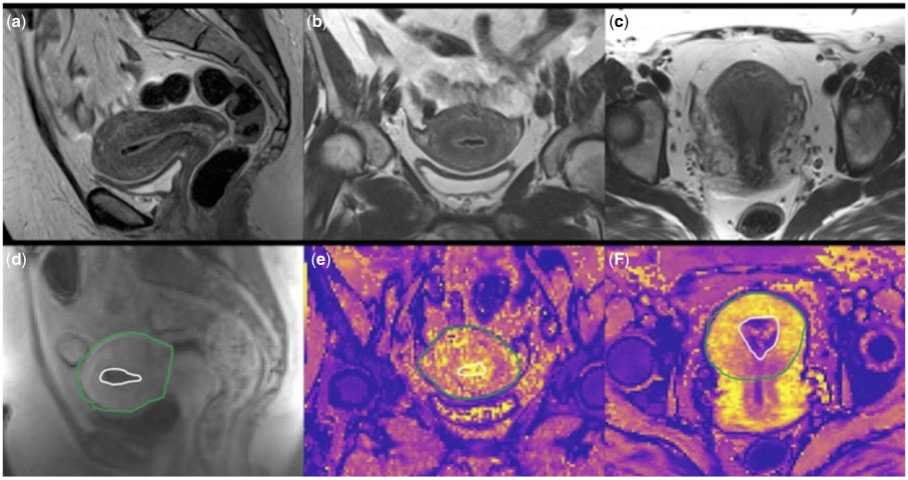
Example images and regions of interest. Top row: T2-weighted (T2w) structural images in the sagittal (a), coronal (b) and axial planes (c), slice locations matching bottom row images for easy reference during outlining. Bottom row: Dynamic contrast-enhanced image (d) showing outlined endometrium (white) and uterus (green). T2* maps in the coronal (e) and axial (f) planes with endometrium and uterus outlined.
